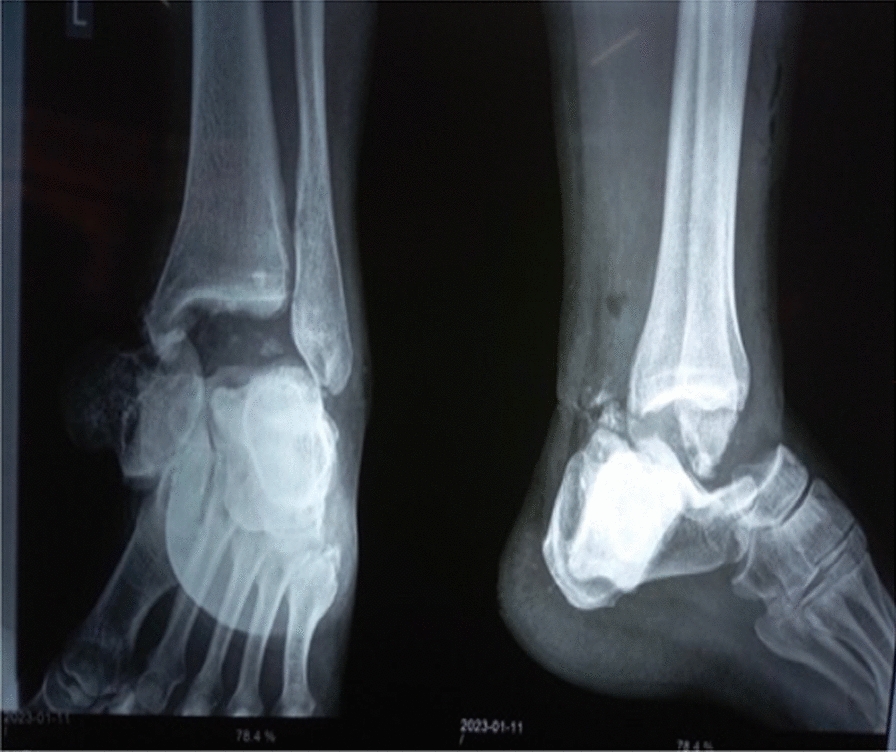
Radiograph showing total talus dislocation
